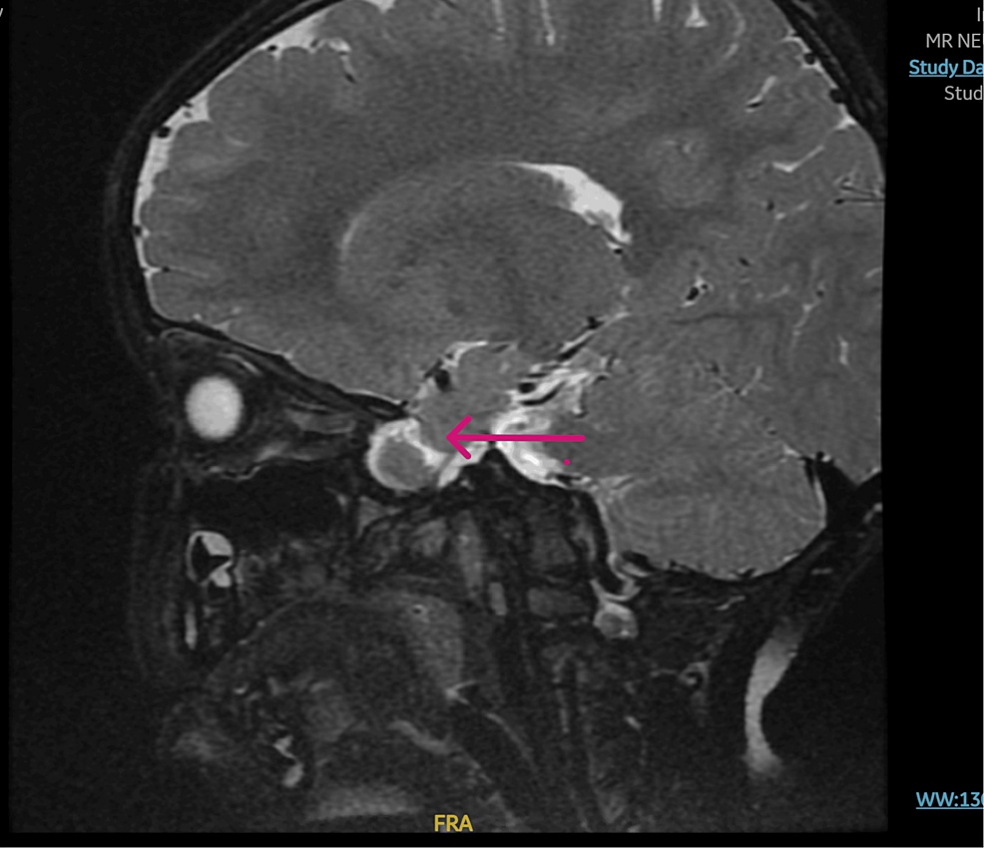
MRI of the brain, sagittal view Subtle bilateral predominately frontal subcortical foci of abnormal susceptibility, likely representing calcifications and suggestive of a remote brain insult with no associated volume loss or other lesions. The brain otherwise demonstrates a normal appearance with preserved volume and signal intensity of the basal ganglia, thalami, brainstem, and cerebellum, and the volume of the pituitary gland is towards the lower limit of normal size with no definite focal lesion, and the pituitary stalk and hypothalamus appear normal. MRI: magnetic resonance imaging
